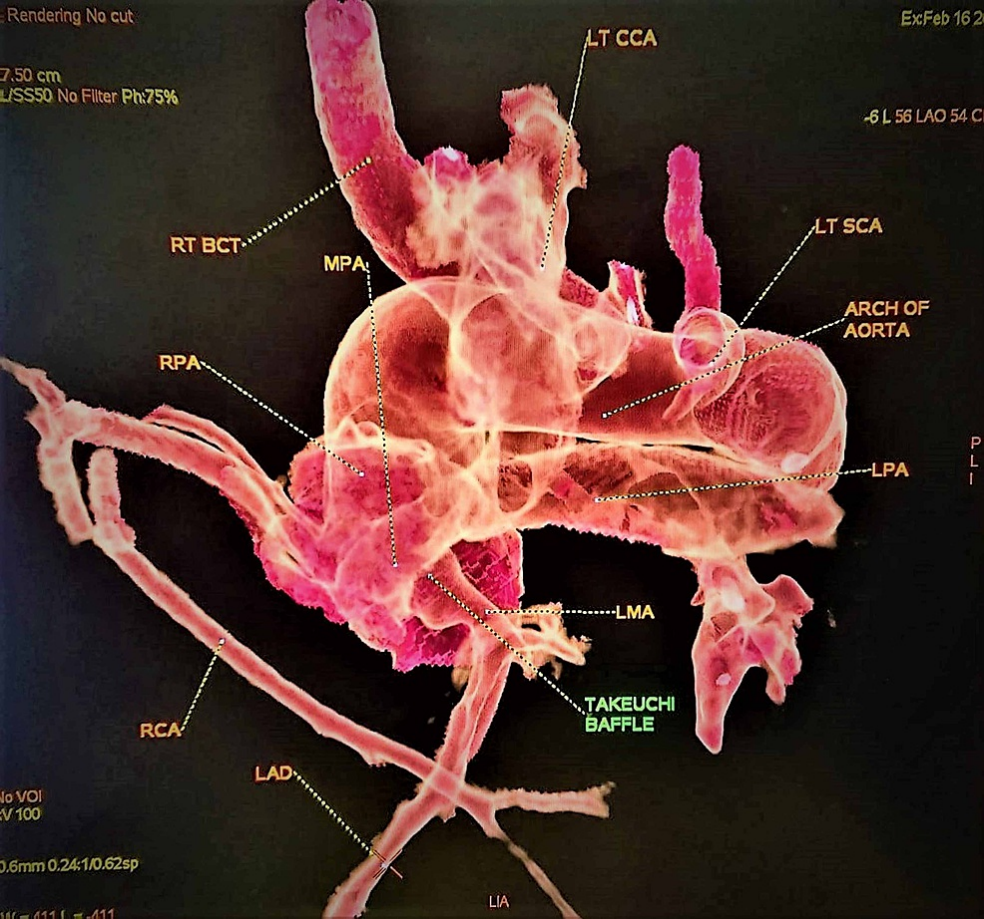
Postoperative Cardiac CT Virtual Reality - Takeuchi Baffle RPA, right pulmonary artery; LPA, left pulmonary artery; RCA, right coronary artery; LAD, left anterior descending; BCT, brachiocephalic trunk; CCA, common carotid artery; SCA, subclavian artery.
